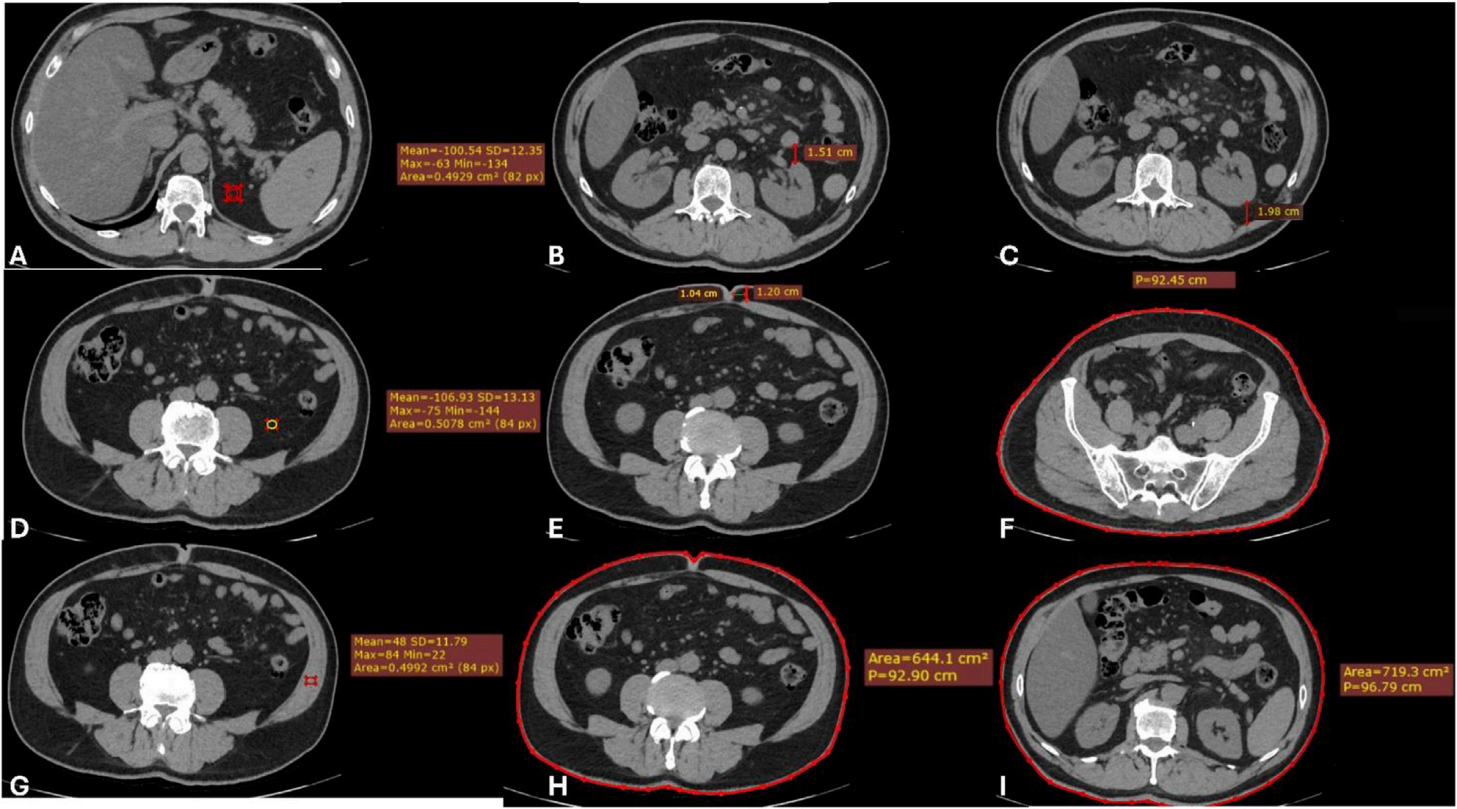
Axial CT images showing the radiological parameters considered. (A) Upper renal fat tissue density (just above the kidney, ROI of 0.5 cm2). (B) Pre-renal fat tissue thickness (at the middle third of the kidney, from the kidney to the bowel). (C) Retro-renal fat tissue thickness (at the middle third of the kidney, from the kidney to the muscle). (D) Lower renal fat tissue density (just below the kidney, ROI of 0.5 cm2). (E) Abdominal wall fat tissue thickness (at 1 cm from the navel). (F) Abdominal circumference (at the antero-superior iliac spine). (G) Oblique muscles density (ROI of 0.5 cm2). (H) Abdominal circumference (at the navel). (I) Abdominal circumference (at the 12th rib).
